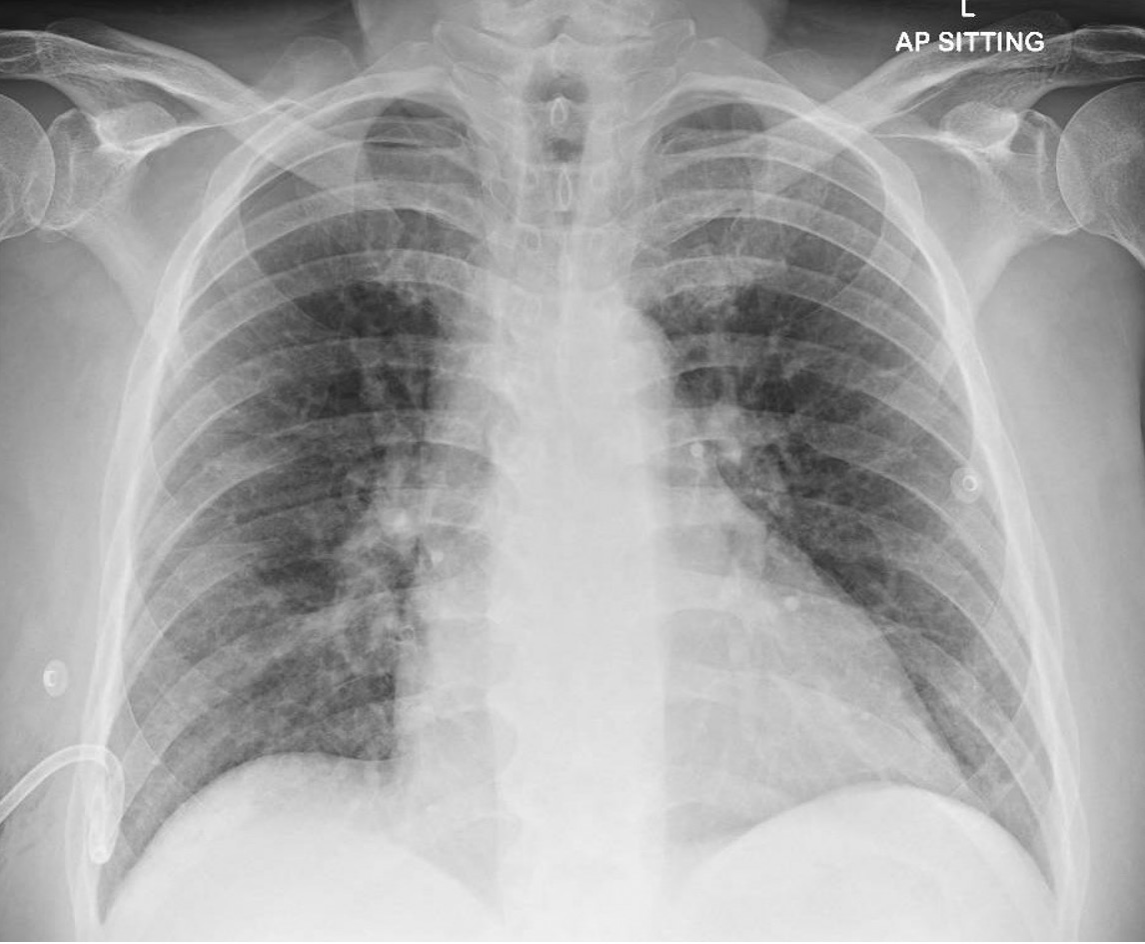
Subsequent chest radiograph demonstrating satisfactory effusion drainage following chest drain insertion.
